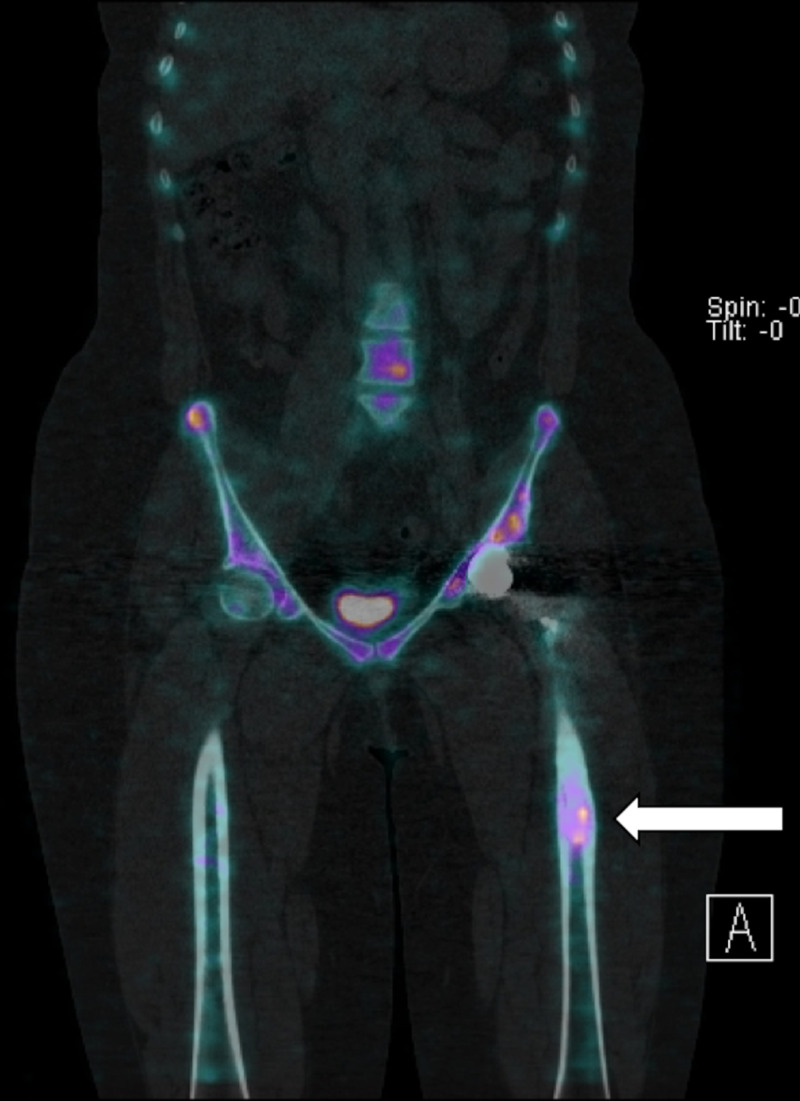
SPECT scan in the coronal view of the trunk and lower limbs. High-intensity uptake is seen in the stem-tip region of the left femur (white arrow). SPECT, single-photon emission computed tomography
